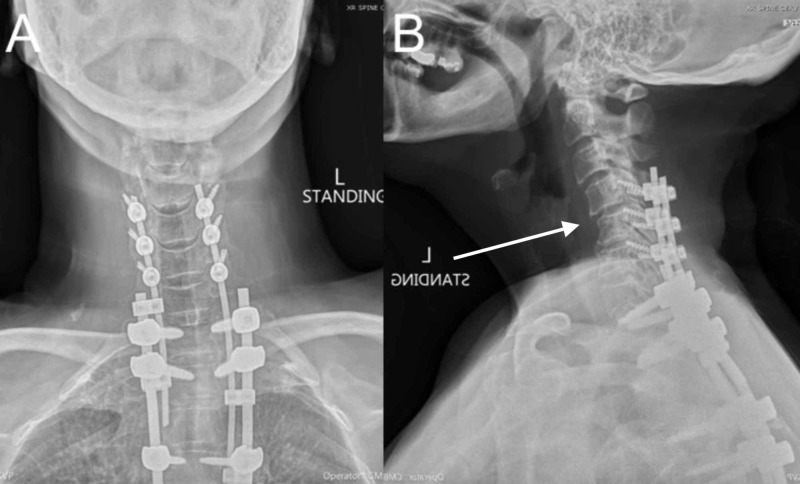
Postoperative cervical spine X-rays Plain cervical spine X-rays, anteroposterior view, showing rostral intact hardware from C4-T7 (A). Plain cervical spine X-rays, lateral view, showing rostral intact hardware from C4-T7 (B).
